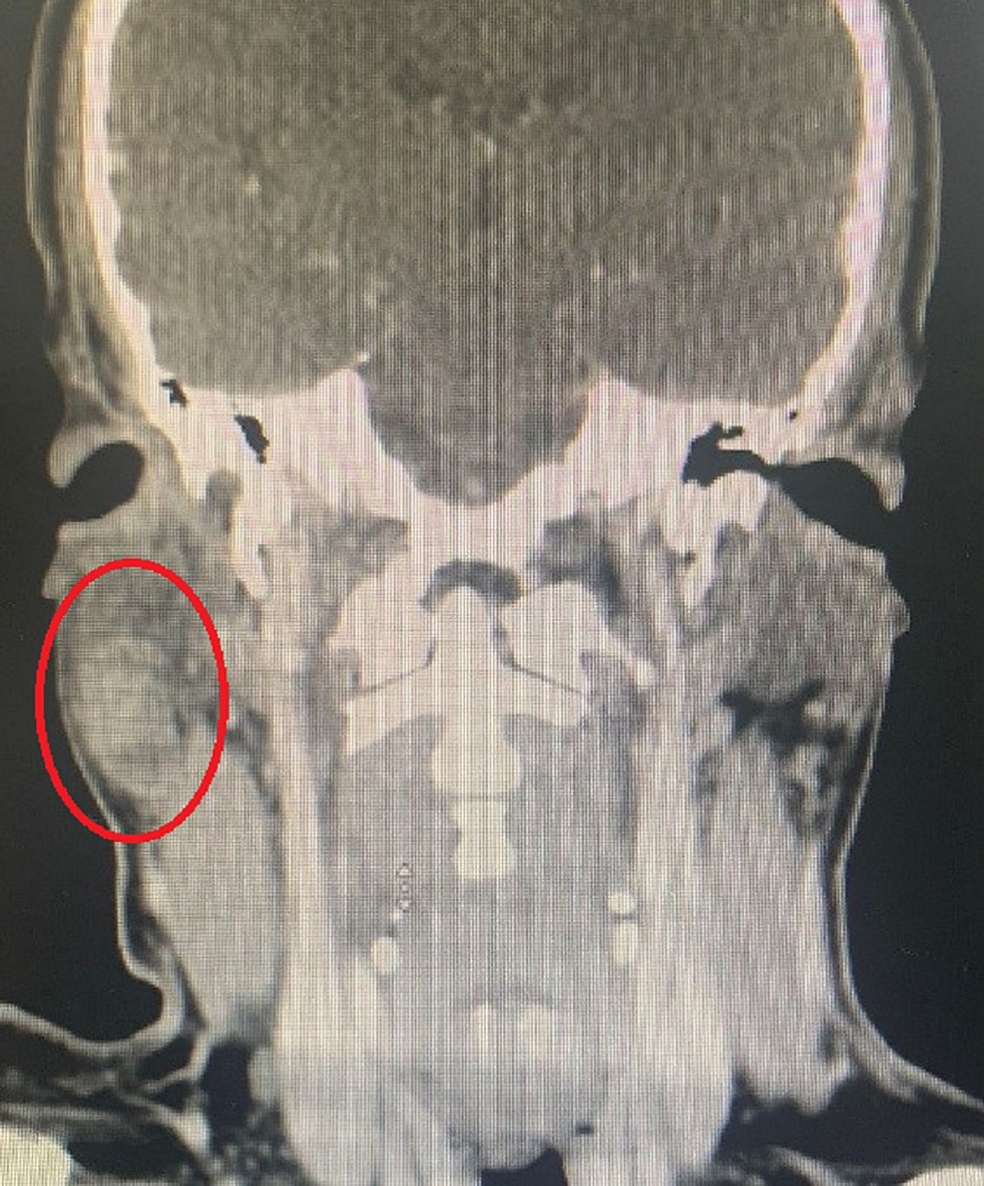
CT scan showing the tumor.
